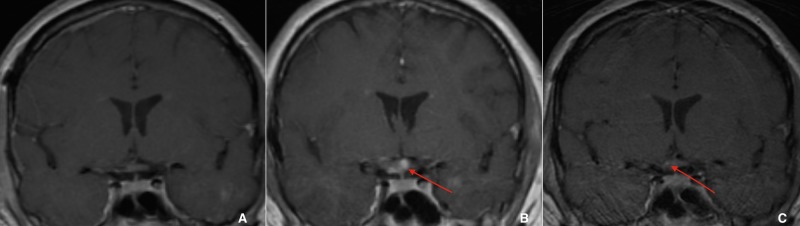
A: Coronal T1-weighted contrast MR image one year postoperatively shows complete resection of the right optic chiasm CM, seen by the absence of hyperintensity with no contrast on enhancement. B: Coronal T1-weighted MR image 32 months postoperatively shows the recurrence of hyperintensity in the right optic chiasm. C: Coronal T1-weighted MR image 39 months postoperatively shows a questionable area of small hyperintensity in the right optic chiasm MR: magnetic resonance; CM: cavernous malformation
